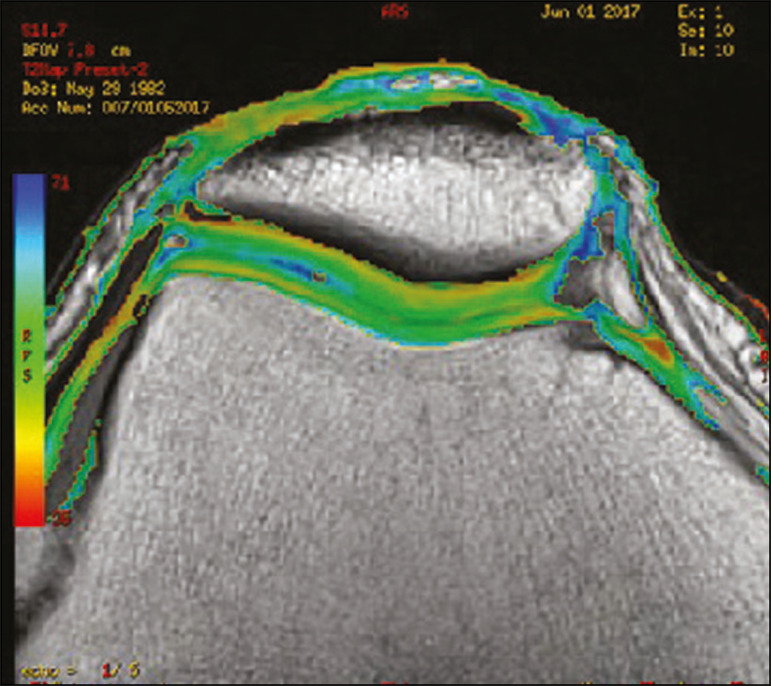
Axial MRI T2 mapping showing normal stratification of the patellofemoral cartilage surface.
